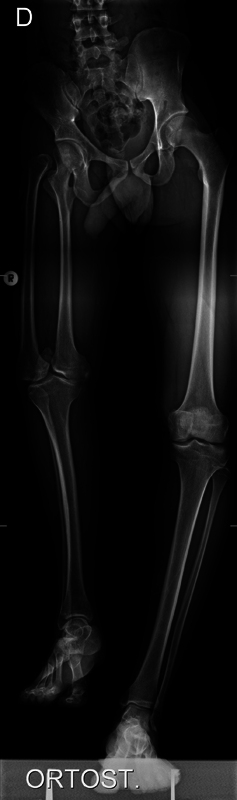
Radiograph in AP view of the lower limbs at 23 years of age. The image shows right-hand valgus femoral neck with normal hip joint, one knee with two separate femoral condyles articulating with the proximal tibia, and a hypolabic patella. The talar dome has a spherical aspect articulating with a concave surface on the tibia, forming a ball-and-socket ankle
